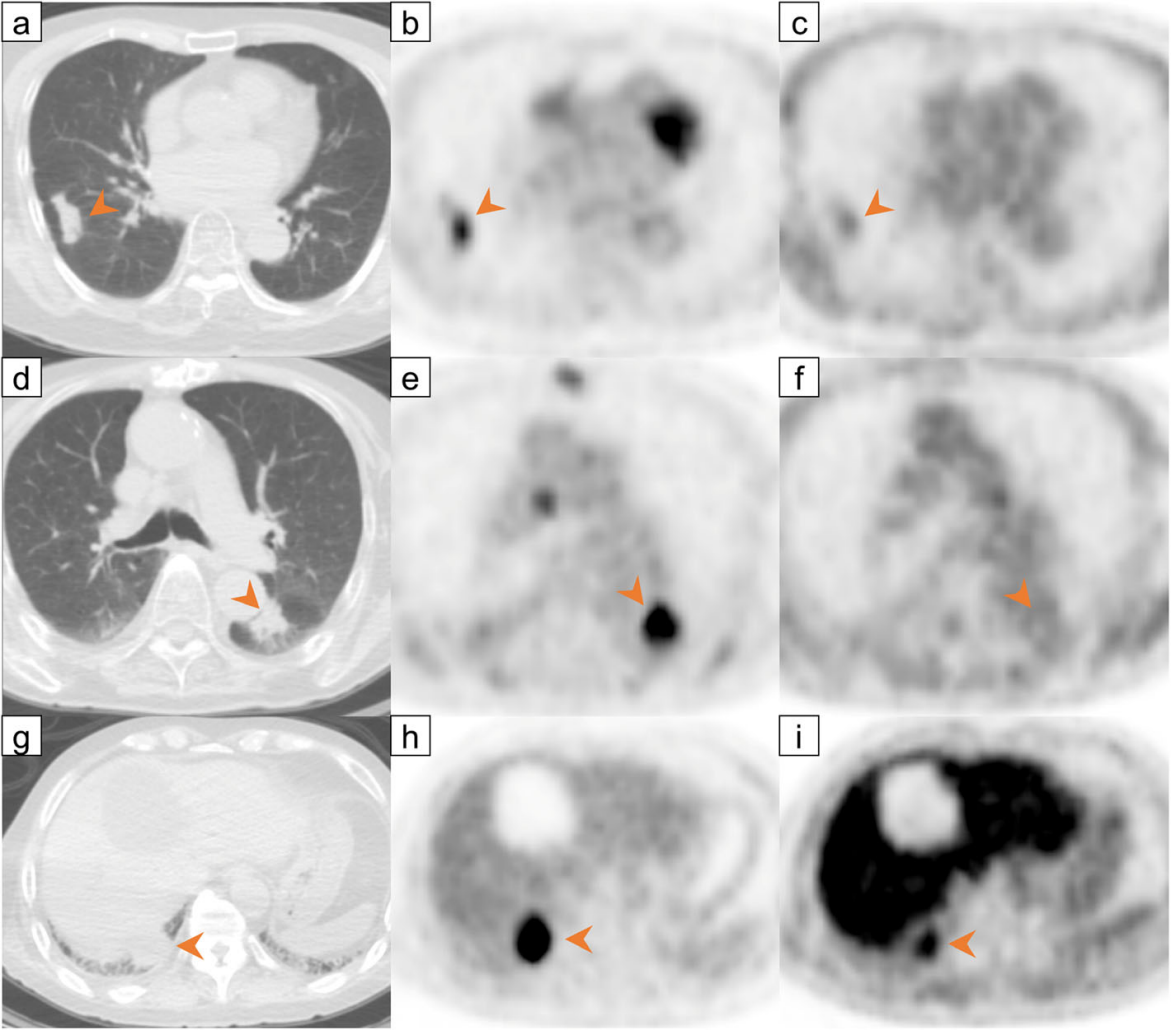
Representative cases of the three patterns of FDG and FMISO SUVmax values. a CT, b FDG PET (SUVmax = 6.02). c FMISO PET (SUVmax = 1.54) image of a patient with pattern A (both low FDG and low FMISO SUVmax) who had no evidence of recurrence on follow-up at 949 days after treatment completion. d CT, e FDG PET (SUVmax = 8.73). f FMISO PET (SUVmax = 1.42) image of a patient with pattern B (high FDG SUVmax and low FMISO SUVmax) who had no evidence of recurrence on follow-up at 540 days after treatment completion. g CT, h FDG PET (SUVmax = 14.04). i FMISO PET (SUVmax = 3.36) image of a patient with pattern C (both high FDG and high FMISO SUVmax) who had recurrence on a mediastinum lymph node on follow-up CT at 195 days after treatment completion
